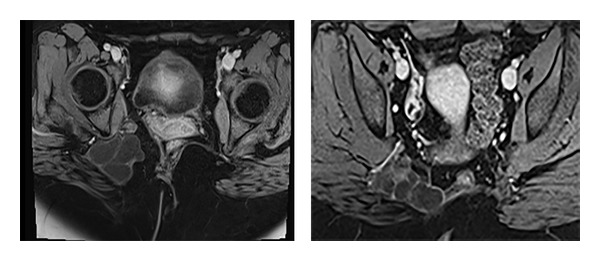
MRI pelvis with recurrent teratoma extending from the retrorectal space and precoccygeal area across midline to the region of right piriformis and deep to right gluteus muscles.
